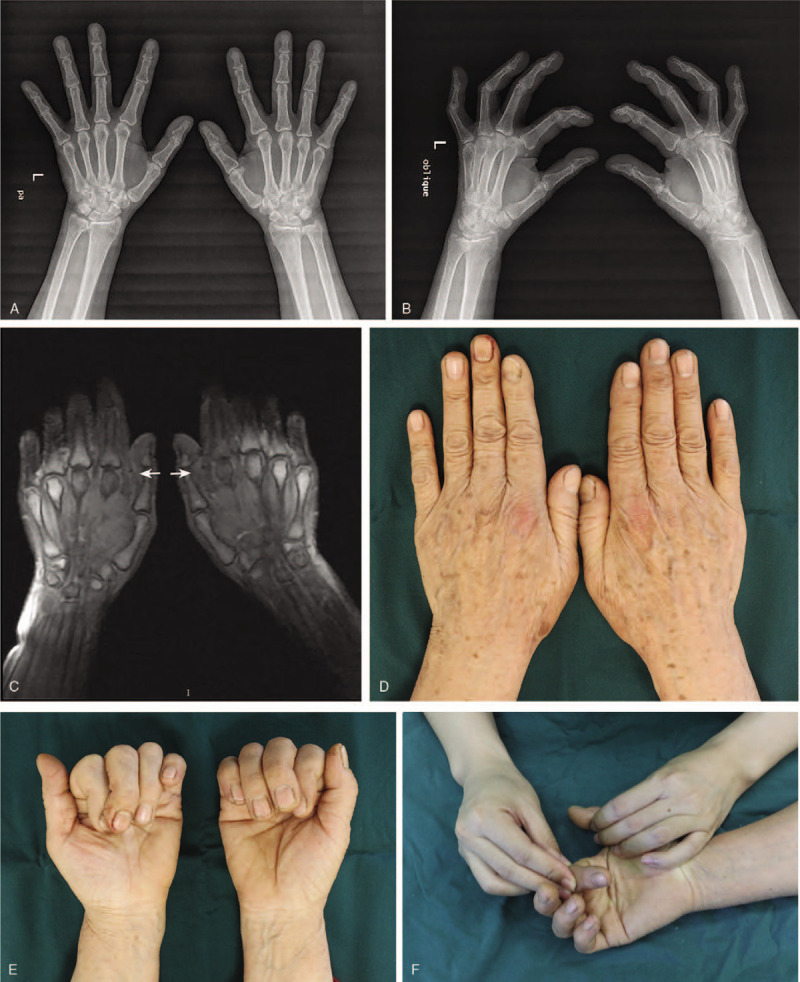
Preoperative imaging. (A and B) MCP joint subluxation of bilateral index fingers on an X-ray. (C) An MRI image confirmed rupture of both RCLs (white arrow). (D) Redness and swelling of the skin at the site of injury. (E) Ulnar deviation and pronation of index finger during active gripping. (F) Passive physical examination. MCP = metacarpophalangeal joint, MRI = magnetic resonance imaging, RCL = radial collateral ligament.
